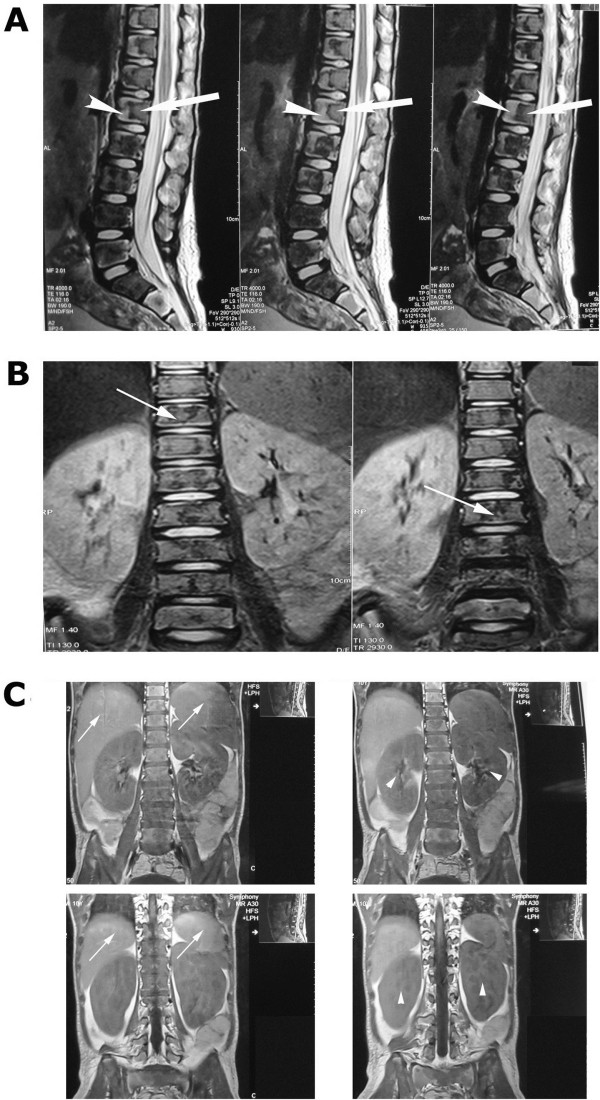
Magnetic resonance imaging of the patient. (A) A side view showing partial loss of normal hydration on hypointense T2-weighted signal intensity of the dorsal and lumbar intervertebral discs, as well as diffuse focal infiltration of the axial bone marrow of the lower dorsal and lumbar vertebrae, causing altered hypointense T1-weighted signal intensity (arrows), in homogeneous hyperintense T2-weighted signal changes (arrowheads). (B) Anteroposterior view. (C) Incidental hepatosplenomegaly (arrows) with bilateral renal enlargement (arrowheads).
